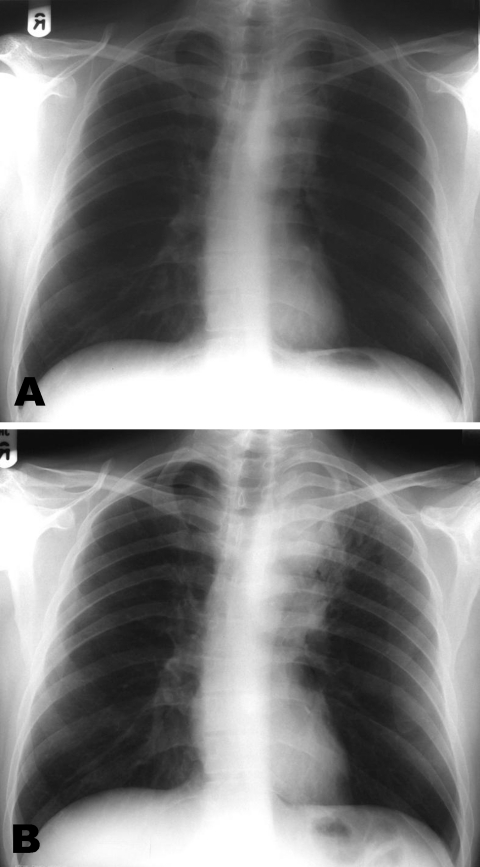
Chest radiographs at initiation of A) highly active antiretroviral therapy (HAART) showing left hilar mass; and B) after 9 weeks of HAART and antituberculosis treatment, suggesting enlargement of the hilar mass consistent with immune restoration disease. (The radiograph has been flipped horizontally to aid comparison).
